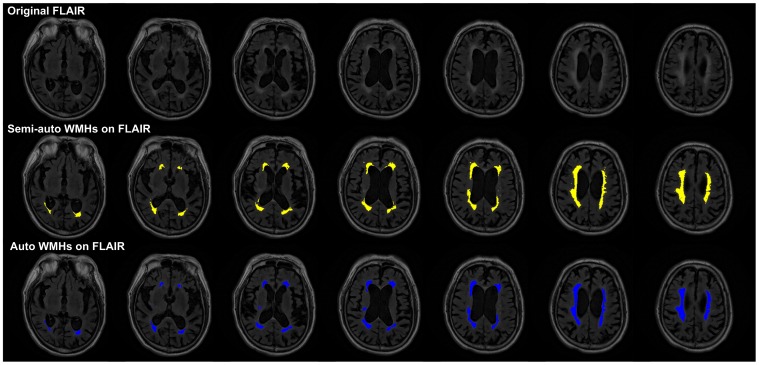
Typical examples of WMHs segmentation. Seven successive axial slices taken from patient #2are shown (upper row: FLAIR image; middle row: yellow regions being the WMHs demarcated by the neurologist; lower row: blue regions being the WMHs demarcated by the proposed algorithm).
